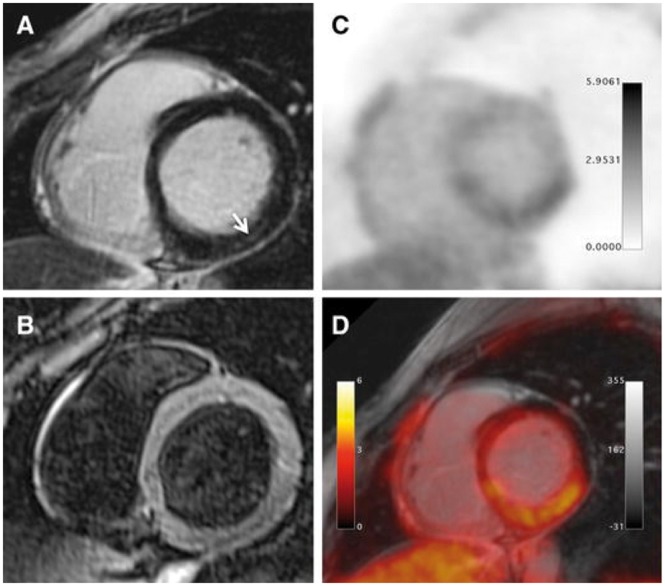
PET-MR images in myocarditis. Inferolateral wall mid-wall fibrosis (MRI) (*A*), homogenous T2 signal intensity (MRI) (*B*). Increased ^18^F-FDG signal in the inferolateral wall (PET) (*C*). Fused PET-MR with increased ^18^F-FDG uptake at the site of inferolateral LGE (*D*). Reproduced with permission from ref. 80.
